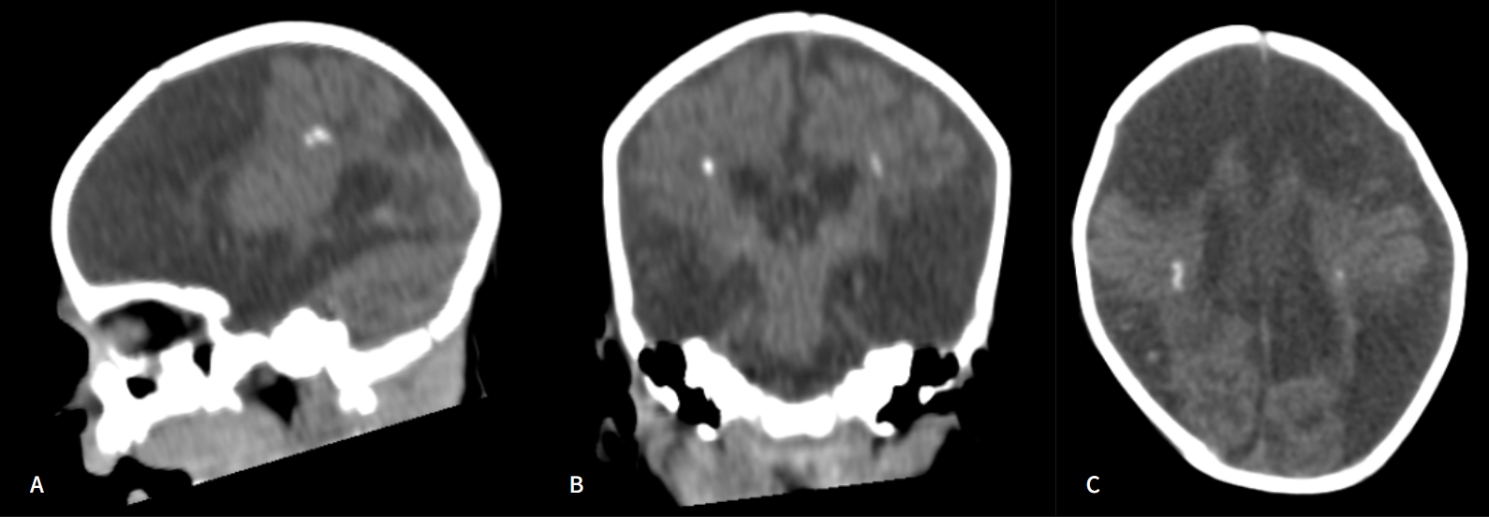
CT scan demonstrating extensive hypodense areas affecting the white matter, multiple areas of parenchymal cystic replacement with sequel-like aspect (cystic leukomalacia), volumetric reduction of the brain and brainstem, and bilateral periventricular foci of calcification; (A) Sagittal, (B) Coronal, and (C) Axial cuts
